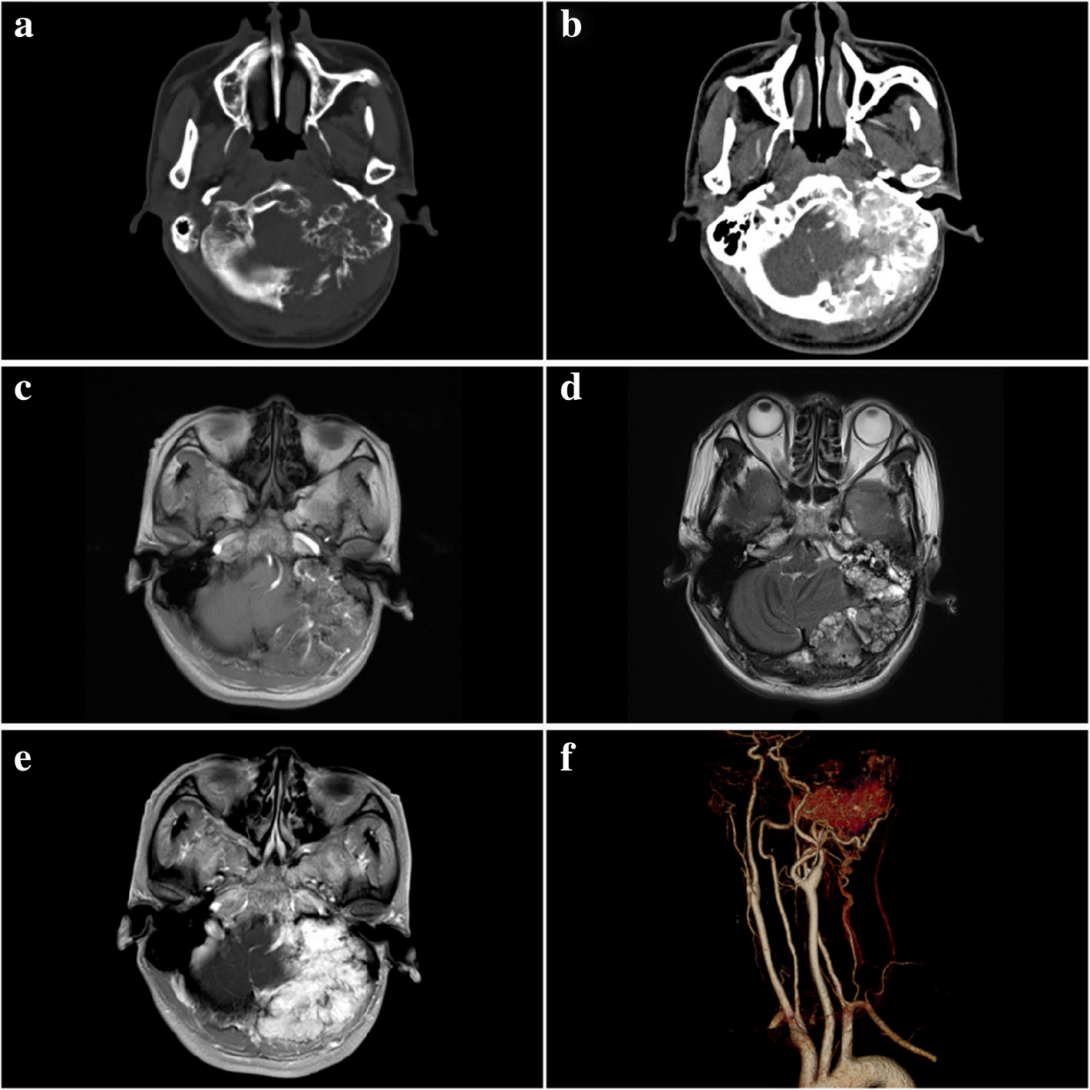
Radiologic characterization of endolymphatic sac tumor. a, b CT in the axial plane demonstrated an expansile lytic lesion of left mastoid process and middle ear extending posteriorly to the posterior cranial fossa (a) with apparent enhancement in contrast imaging (b). c–e MR in the axial plane showed the lesion to be uniformity hypo-signal on the T1-weighted (c) and high-low mixed signs on the T2-weighted (d), and obviously enhanced with contrast (e). f Three-dimensional computerized tomographic angiography ( 3D-CTA ) manifested main blood supply of lesion was from the external carotid artery and branches of the subclavian artery
